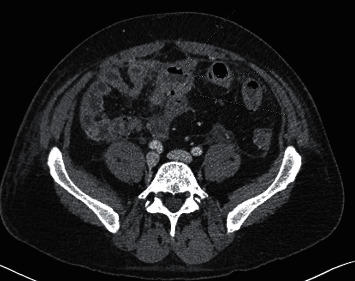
Axial CT enterography image of the lower abdomen shows persistent inflammation of a short segment of the distal ileum. This segment of the ileal wall is thickened and edematous with minimal surrounding edema.
